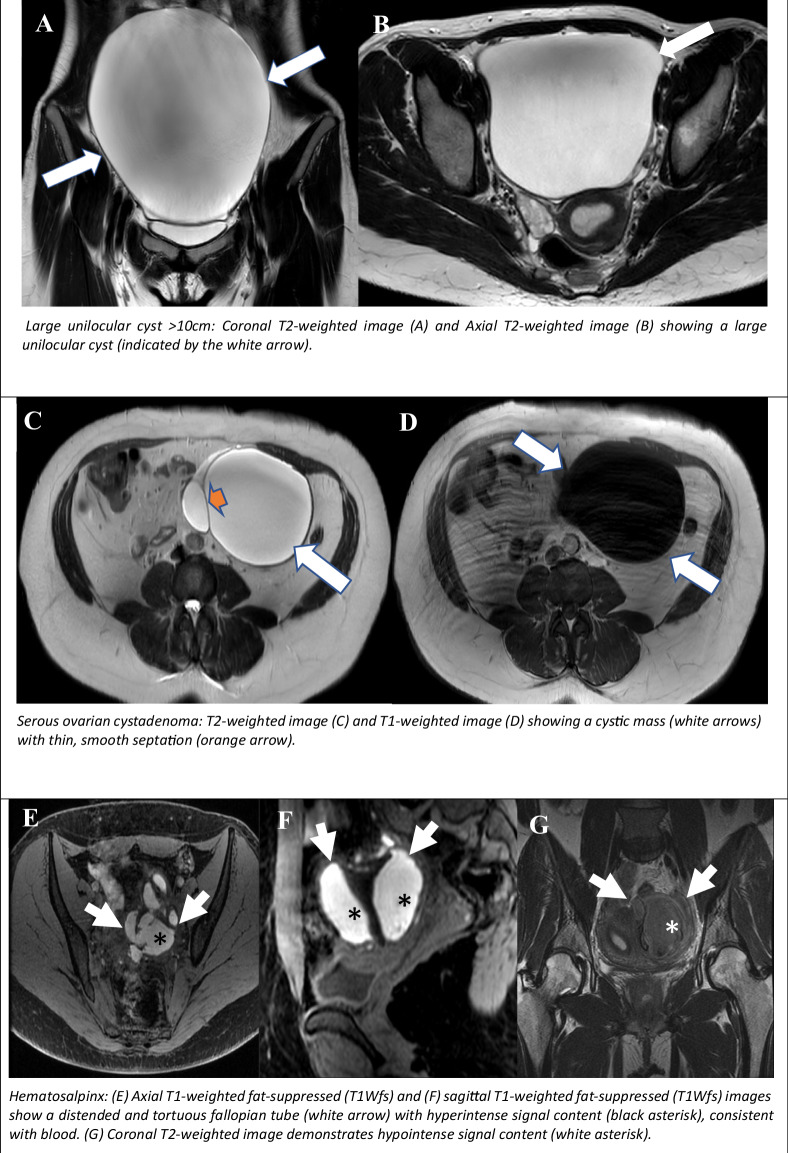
O-RADS MRI 3: cystic ovarian lesions with low risk for malignancy. Large unilocular cyst > 10 cm: coronal T2WI (A) and axial T2WI (B) showing a large unilocular cyst (indicated by the white arrow). Serous ovarian cystadenoma: T2WI (C) and T1-weighted image (D) showing a cystic mass (white arrows) with thin, smooth septation (orange arrow). Hematosalpinx: (E) axial T1-weighted fat-suppressed (T1Wfs) and (F) sagittal T1-weighted fat-suppressed (T1Wfs) images show a distended and tortuous fallopian tube (white arrow) with hyperintense signal content (black asterisk), consistent with blood. G The coronal T2WI demonstrates hypointense signal content (white asterisk)
